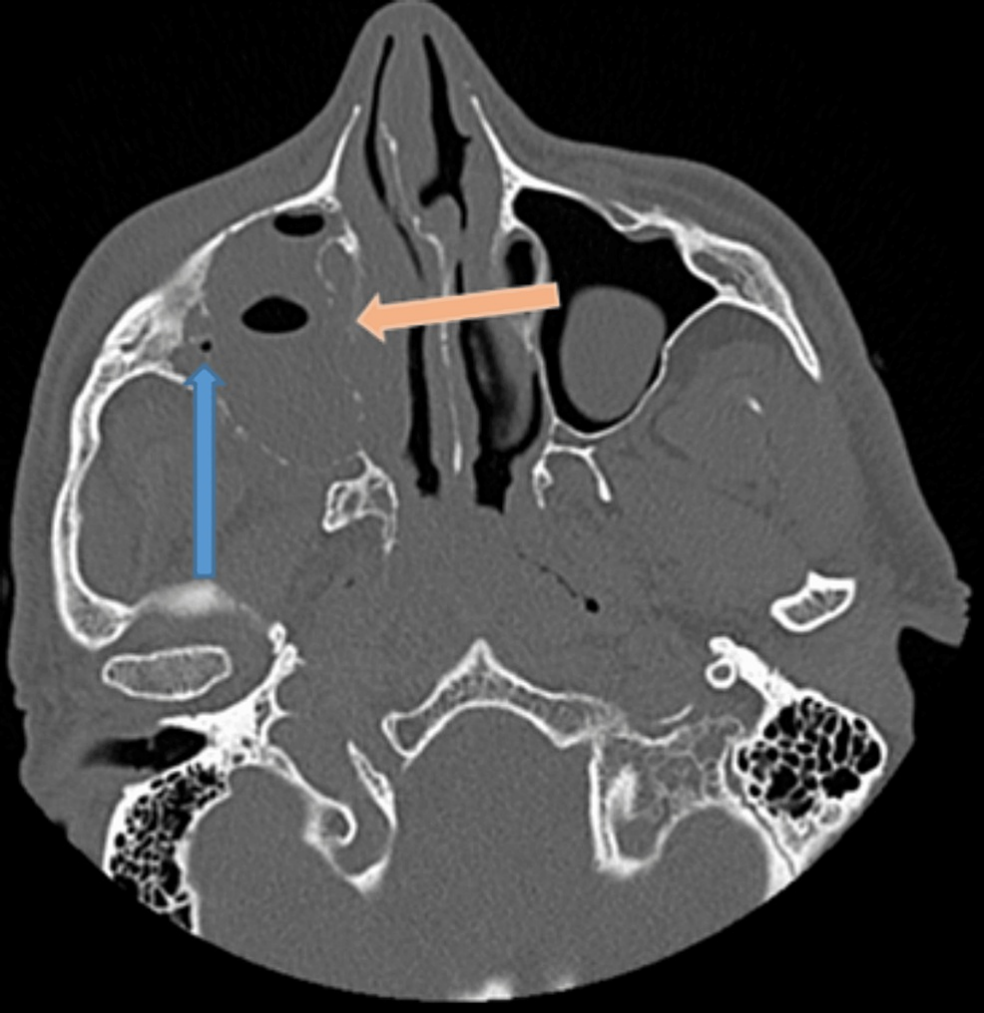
CT sinus – axial image Sub-total opacification of the right maxillary sinus with thinning and dehiscence of the medial and lateral sinus walls (orange arrow). There is a suggestion of an intra-sinus IOC with bony dehiscence (blue arrow).
